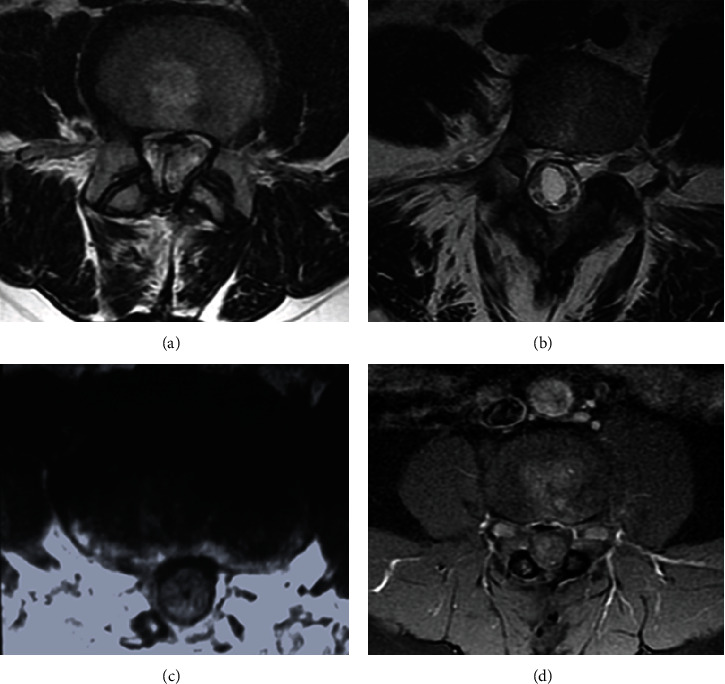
Axial view of lumbar MRI showing different patterns of arachnoiditis. (a, b) T2 sequence showing clumped-up roots in the thecal sac. (c, d) T1 shows an axial view of the lumbar spine in the final stage of the inflammatory response and a swelling mass that occupies the dural sac.
